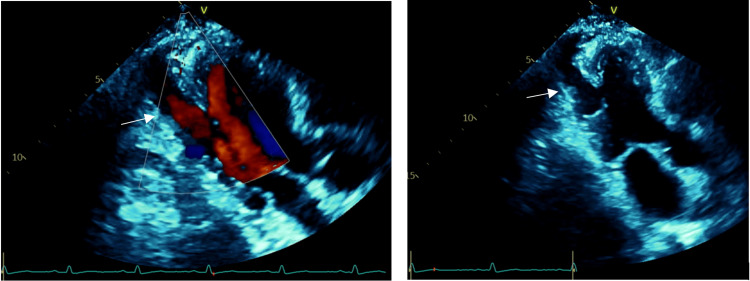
Echocardiogram Echocardiogram of three-chamber view with color compare showing the left ventricular diverticulum - arrow.
